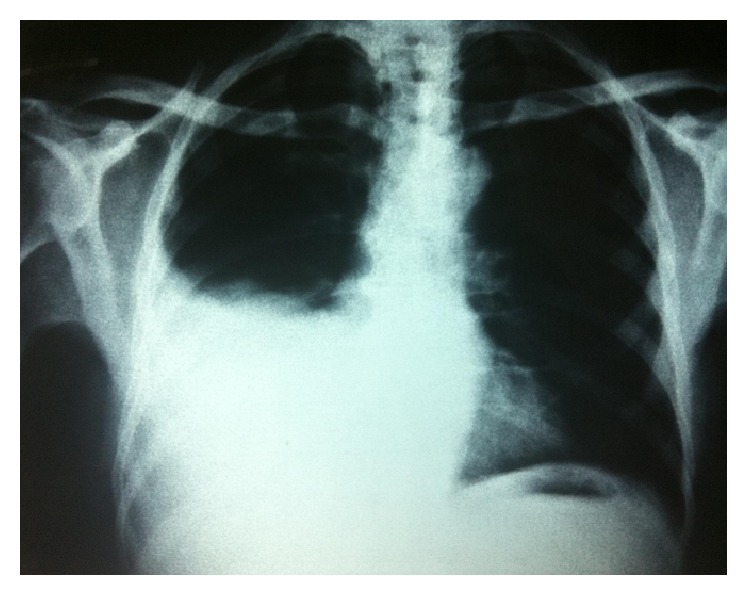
Chest X-ray showing right sided moderate pleural effusion (hepatic hydrothorax).
